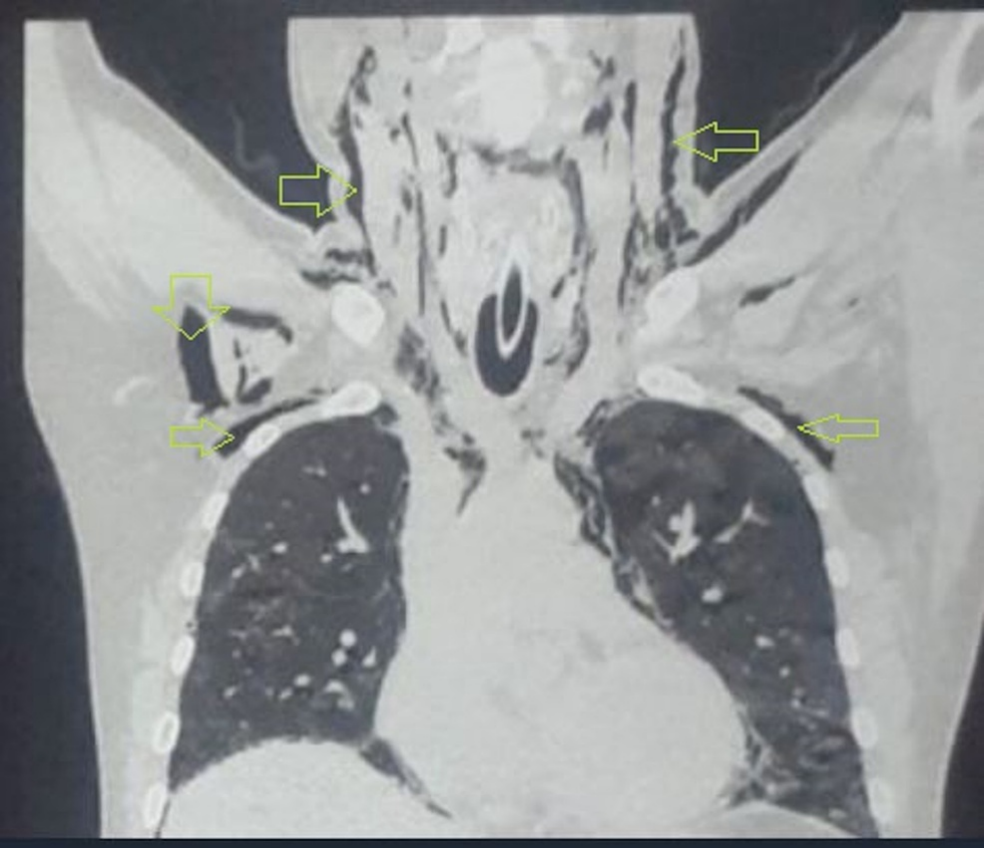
Coronal view of CT showing subcutaneous emphysema (shown by yellow arrows)
